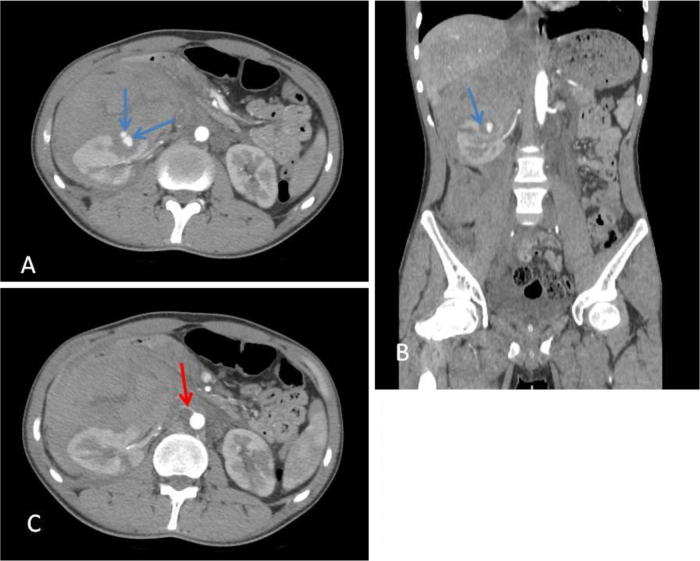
Axial (A) and coronal (B) views of post-contrast-enhanced computed tomography scan show partially thrombosed pseudoaneurysm (blue arrows) and accessory right renal artery (red arrow).
